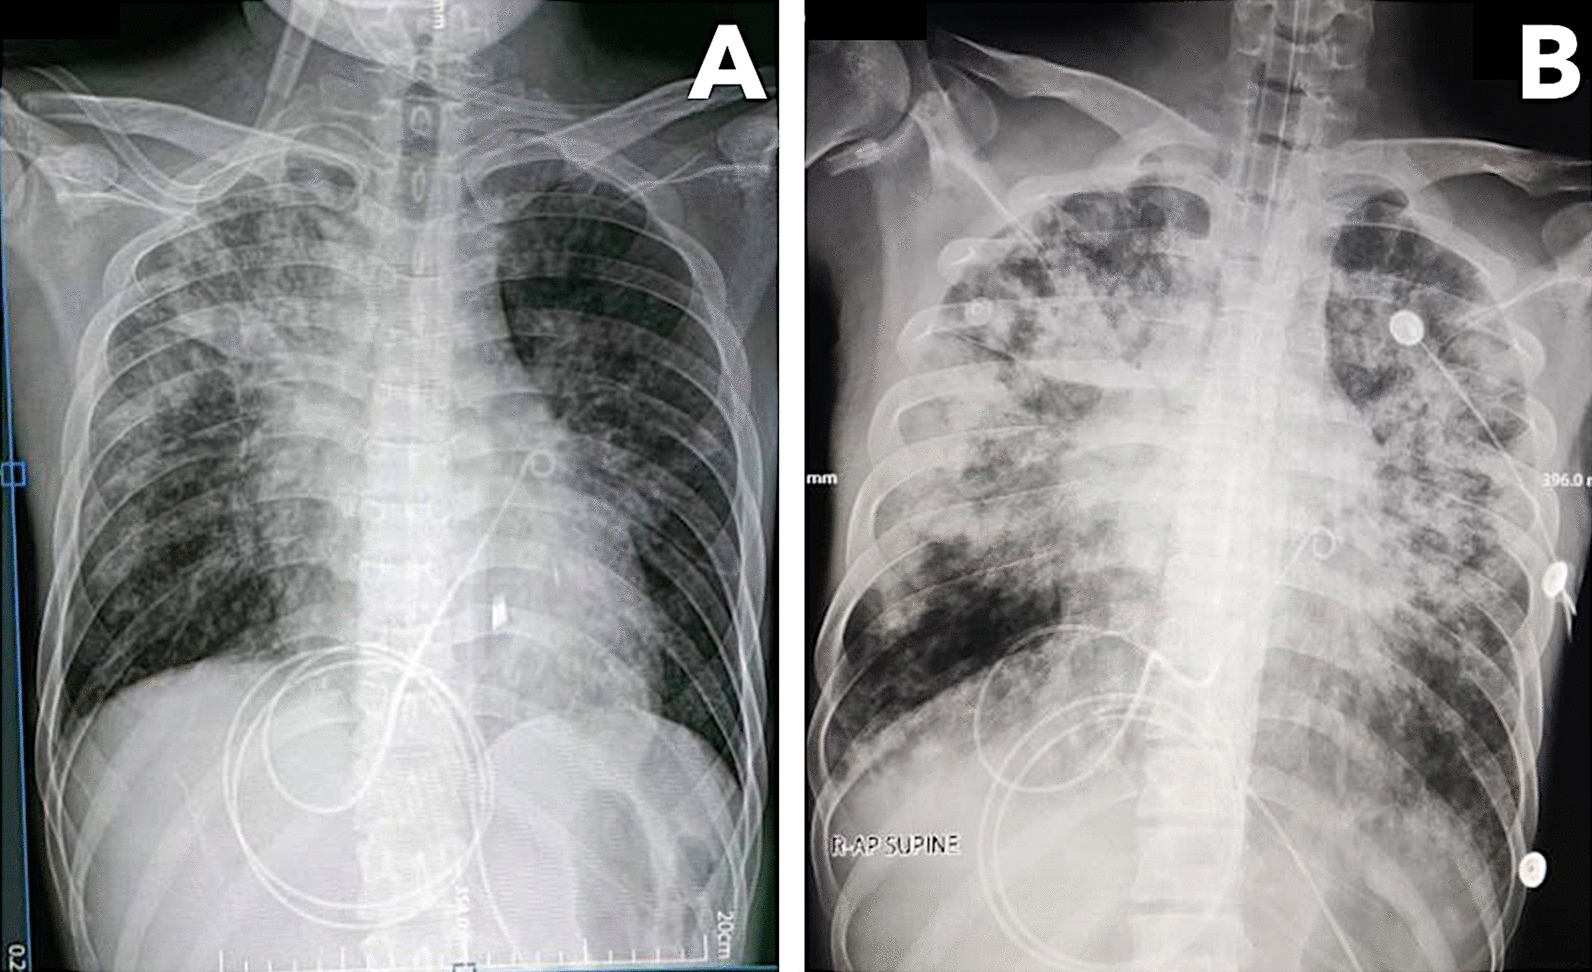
Chest radiographs taken after pericardiocentesis with visible reduction in the size of the cardiac silhouette (A) and taken 12 days after with progression of air bronchograms and fibroreticular infiltrates in both lung fields (B)
